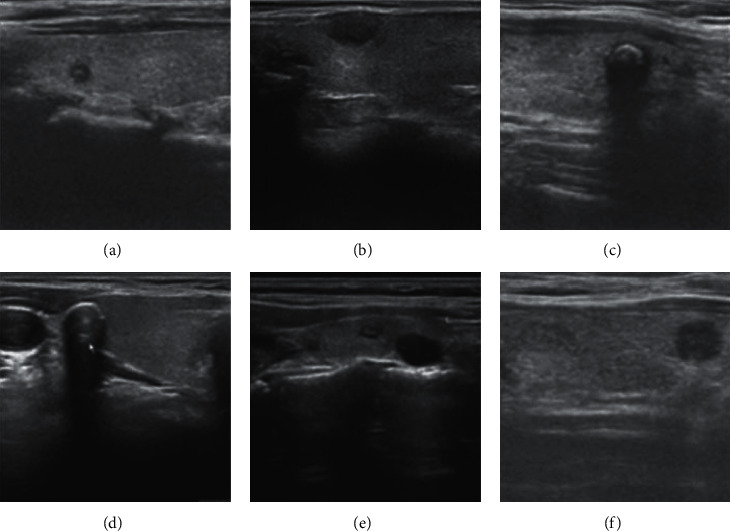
Ultrasound image of benign cases. (a) Round shape; (b) smooth margin; (c) coarse calcification; (d) hyperechogenicity; (e) cystic appearance; (f) clear boundary.
